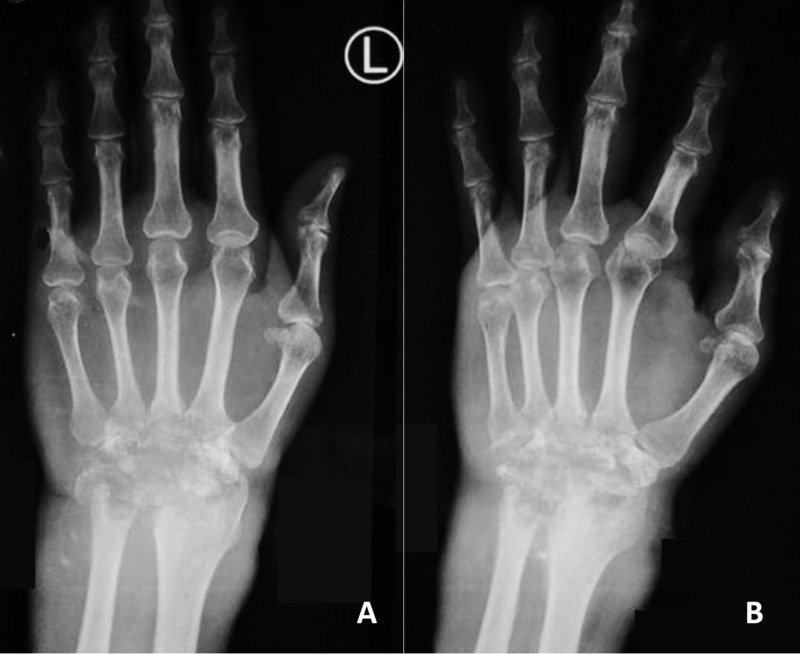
Plain radiograph of the wrist. (A) Anteroposterior view. (B) Oblique view.
